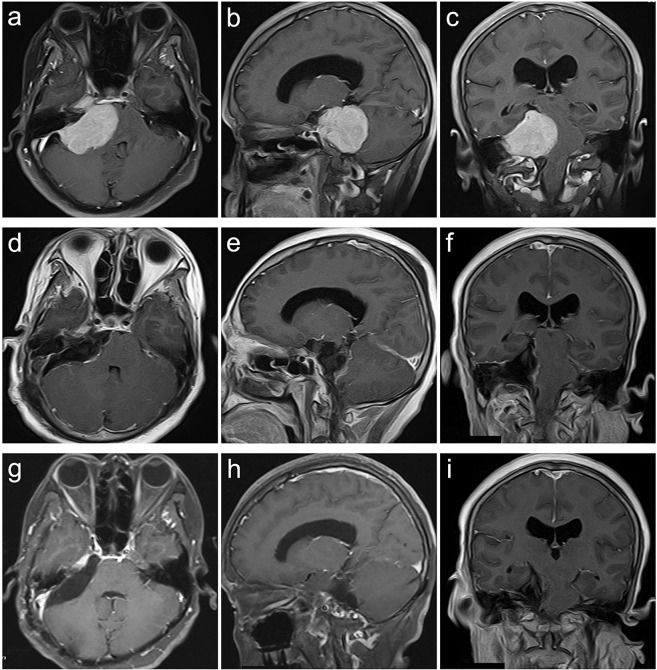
Petroclival type case. A 47-year-old female presented with headache, dysphagia, bucking, hearing impairment and ataxia for 36 months. The preoperative KPS score was 60. She was achieved GTR with the RTTA of palsy in CN V VI,VI and VII and gastric intubation. The postoperative KPS score was 50. With a follow-up of 24 months, she participated in normal activities and had a KPS score of 80 without recurrence. (a–c) Preoperative MRI T1 contrast axial, sagittal, and coronal images. (d–f) Postoperative MRI T1 contrast axial, sagittal, and coronal images. (g–i) Follow-up MRI T1 contrast axial, sagittal, and coronal images.
